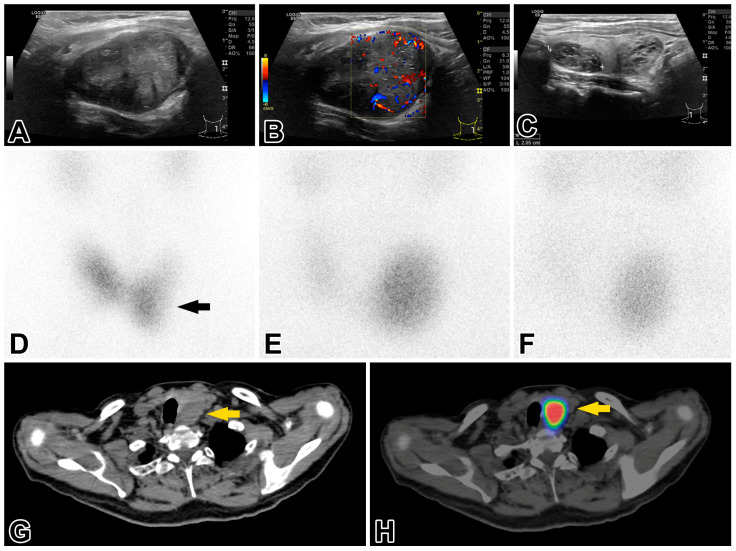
Neck ultrasound revealed (A) a large, hypoechoic, slightly irregularly shaped but sharply contoured, heterogeneous, solid nodule in the caudal half of the left thyroid lobe (EU-TIRADS 3); (B) the nodule exhibited enhanced central and peripheral vascularization; and (C) there were several smaller EU-TIRADS 2 nodules in the right lobe, displaying purely spongiform structures. Thyroid scintigraphy (D) showed a contour-deforming activity defect in the middle lower third of the left lobe, corresponding to a cold nodule (arrow). Parathyroid scintigraphy demonstrated a ^99m^Tc-MIBI-enriched nodule in the left lobe corresponding to a large parathyroid neoplasm. (E) The early phase (10 min) and (F) late phase (120 min) parathyroid SPECT/CT. (G) Axial slices of native (low-dose) CT images show an inhomogeneous hypodense lesion of 40 × 32 mm^2^ axial diameter in the lower half of the left thyroid lobe, not sharply demarcated from it, with a dislocation of the trachea to the right (yellow arrow). (H) The marked lesion displays an intensely enhanced radiopharmaceutical uptake in SPECT/CT fused images.
